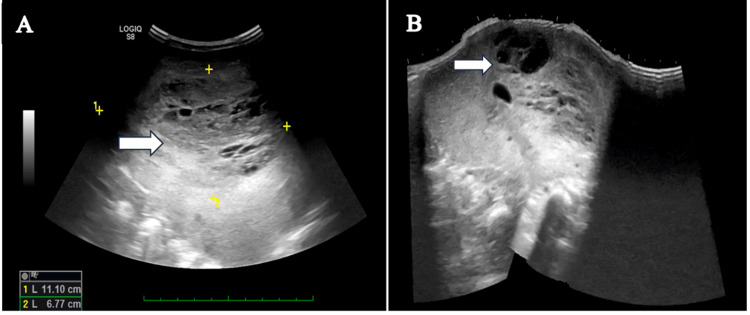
Ultrasound image showing (A) a large multilocular solid cystic lesion (white arrow) almost completely replacing liver parenchyma and (B) multiple internal cystic areas (white arrow) giving Swiss-cheese appearance.
